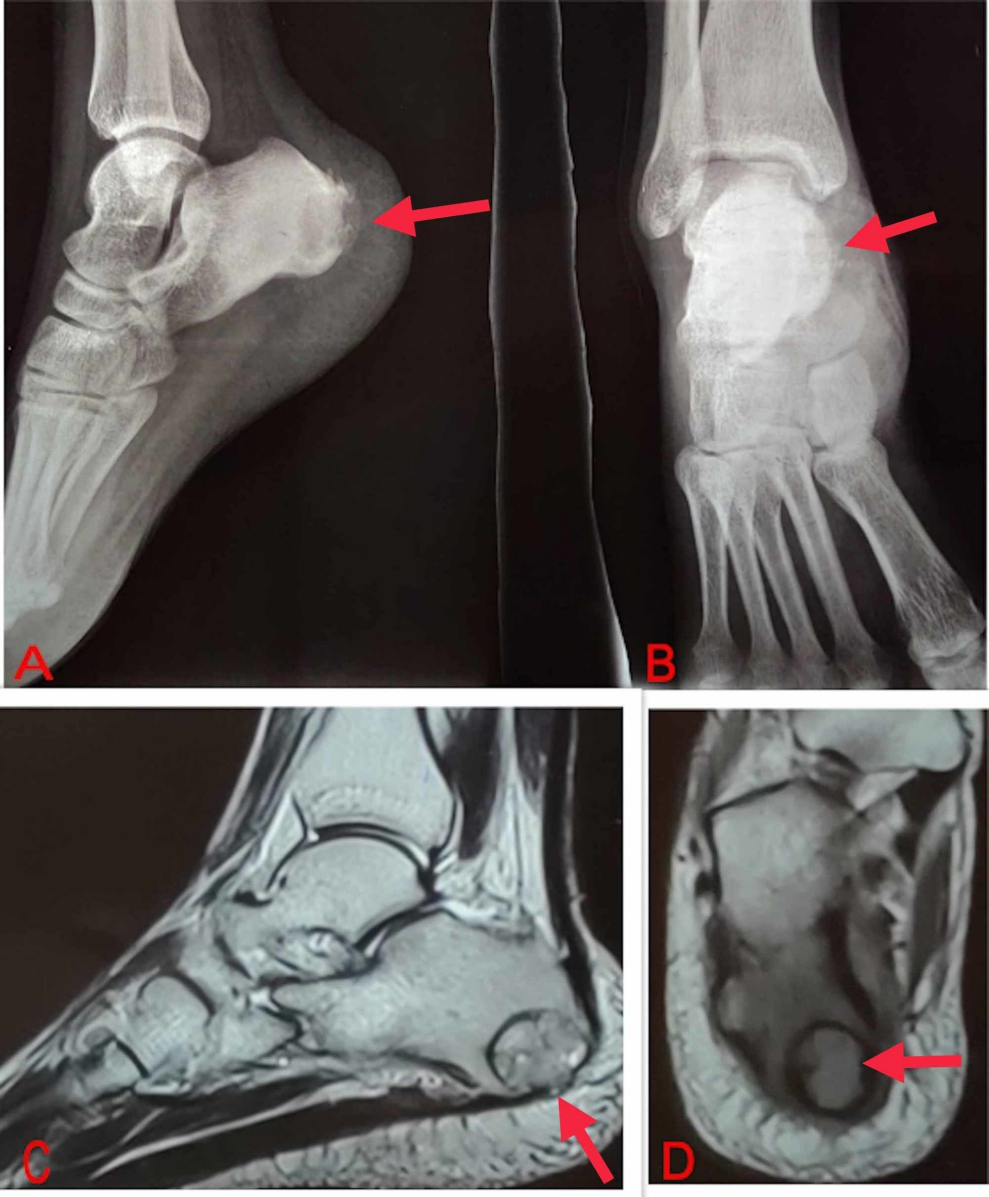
Preoperative radiographic assessment of the lesion. An expansile, eccentric, lytic lesion with non-sclerotic rim in the right calcaneus on X- rays (A, B); a well-defined hyperintense lesion with cystic areas abutting the posterior and medial surfaces of the calcaneus with hypointense margins and narrow zone of transition on MRI (C, D).
